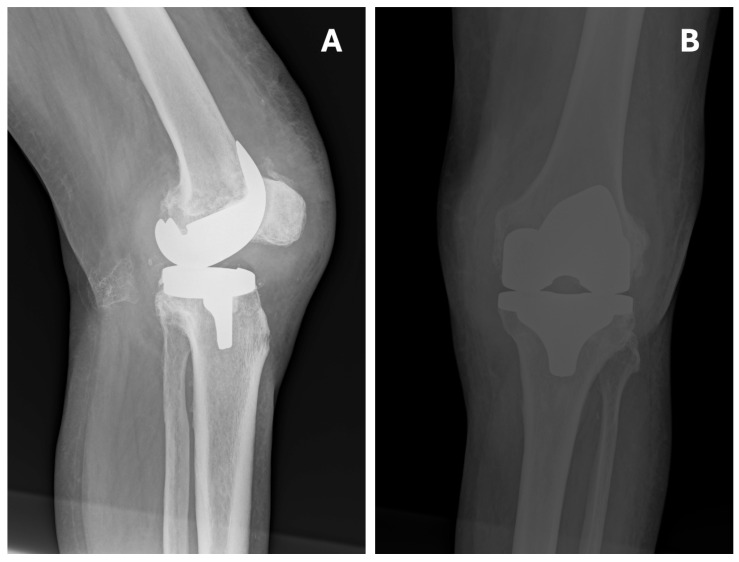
Postoperative X-ray after repositioning. (A) Lateral plane. (B) Anteroposterior (AP) plane. The correct axis is presented in both the AP and lateral views.
